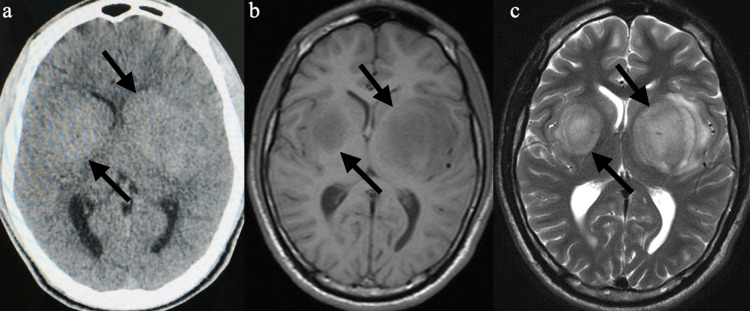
CT and MRI (a) Noncontrast computed tomography scan showing bilateral hyperattenuating lesions in the basal ganglia (black arrows); (b) Axial T1-weighted magnetic resonance image with low-intensity lesions, larger on the left, with lateral ventricle compression (black arrows); (c) Axial T2-weighted magnetic resonance image with hypersignal (black arrows)
